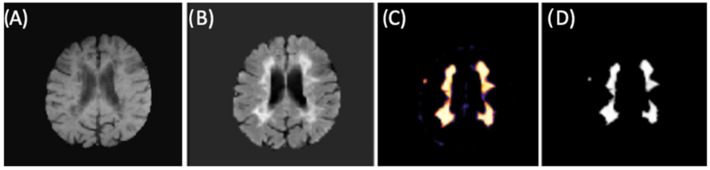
CNN example for lesion segmentation. (A) T1-w image after preprocessing and brain extraction. (B) FLAIR image after preprocessing and brain extraction (second CNN input). (C) Lesion memberships. (D) Binary mask by thresholding the probabilistic memberships.
